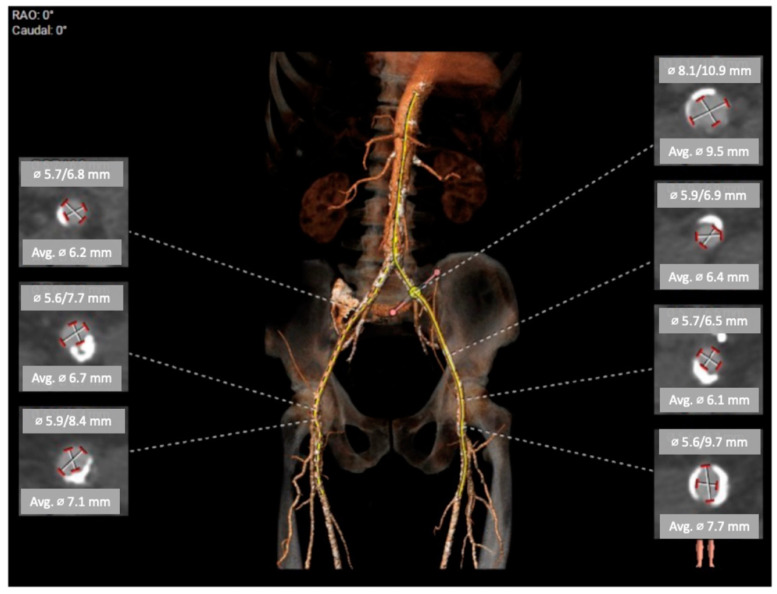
CTA with 3D reconstruction—access vessels.
